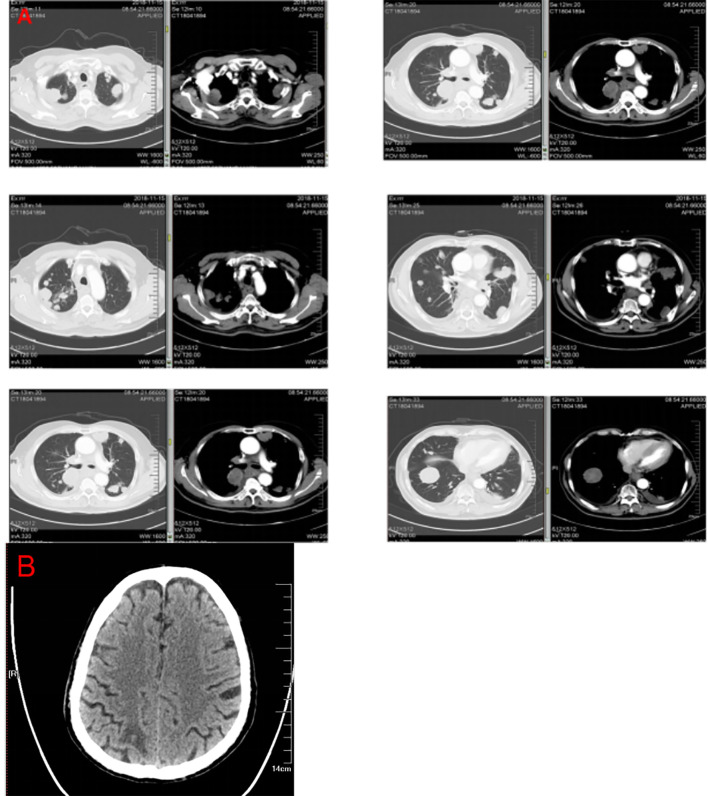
Computed tomography images from the patient with esophageal squamous cell carcinoma. Computed tomography imaging revealed an expansile intraluminal esophageal mass and lung masses (A). The mass observed in brain computed tomography (B).
